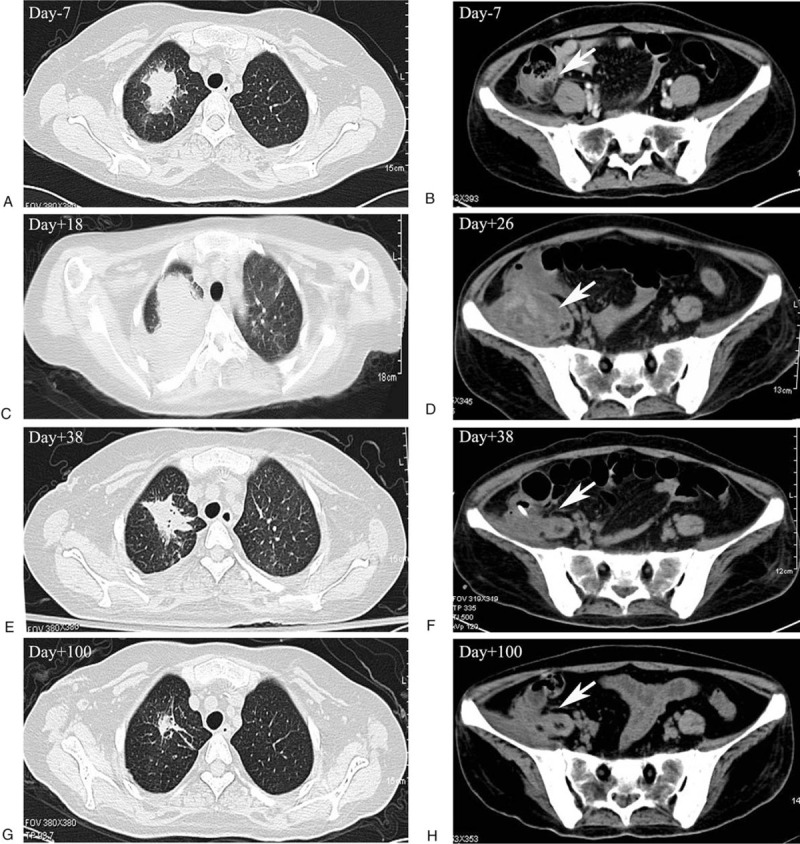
Dynamic images of acute perforated appendicitis and pneumonia before and after transplantation (A,C,E,G) Chest high resolution computed tomography images on day-7, day+18, day+38 and day+100 respectively, demonstrating the dynamic changes of the lesion in the right upper lung. (B,D,F,H) abdominal computed tomography images on day-7, day+26, day+38 and day+100, respectively, demonstrating the dynamic changes of acute appendicitis.
